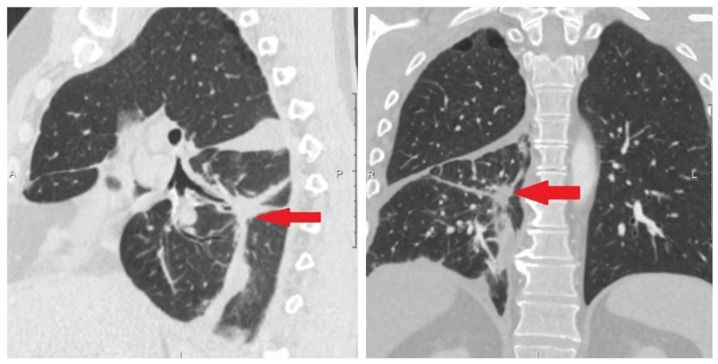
Protocol code 10: CT scan of the chest from 13 May 2021. Fibrous changes at the site of tumor tissue (indicated by arrow).
